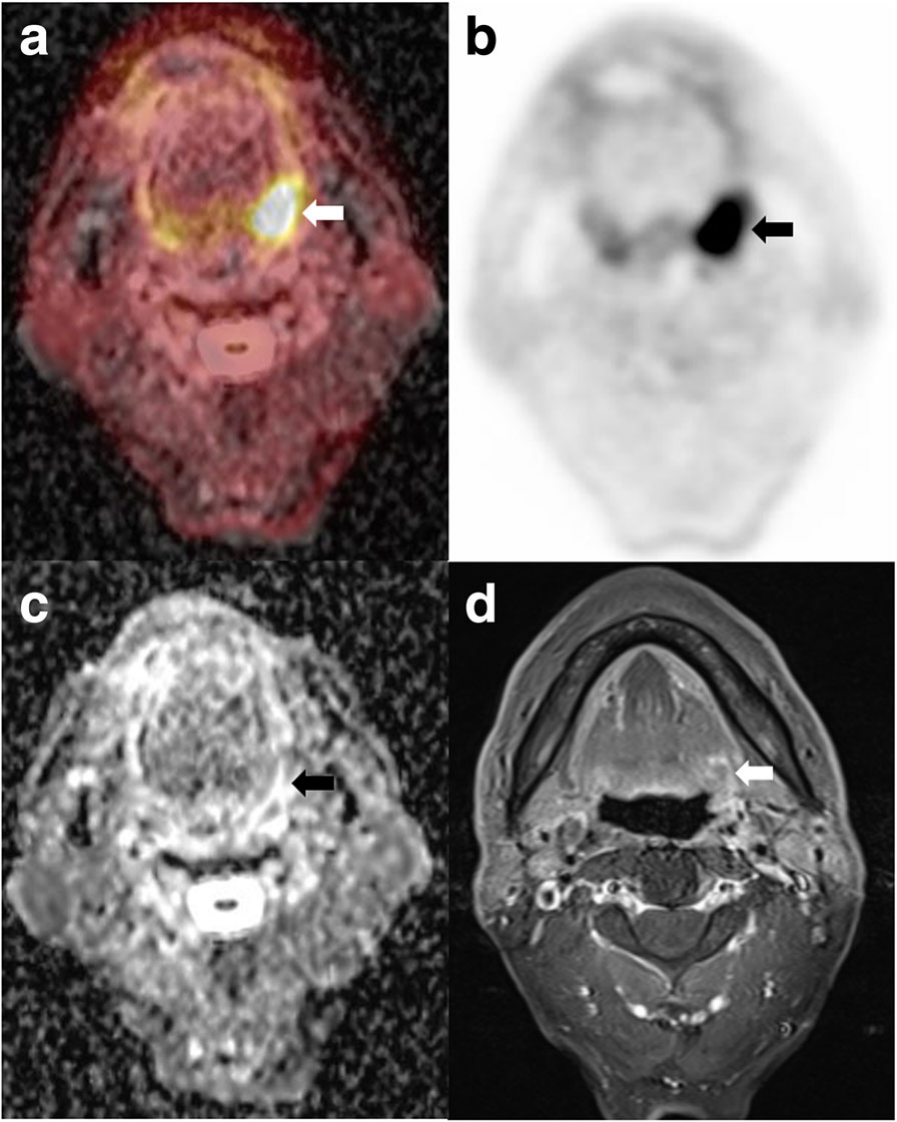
Case of tonsillar cancer with no evidence of disease predicted by combined PET/MRI parameters but treatment failure predicted by PET parameters. A 66-year-old male underwent preoperative simultaneous ^18^F-FDG PET/MRI due to a 3.8-cm-sized left tonsillar cancer. a Fusion image of regional PET with ADC map and b regional PET images showed left tonsillar mass with hypermetabolism (arrows). c ADC map image demonstrated left tonsillar mass with diffusion restriction (arrow). d Gadolinium-enhanced T1 axial image revealed mass with peripheral enhancement (arrow). ADCmean (1443.85), (SUVmax/ADCmean) × 1000 (10.37), (MTV/ADCmean) × 1000 (10.69), and (TLG/ADCmean) × 1000 (57.53) predicted no evidence of disease; however, SUVmax (14.97), MTV (15.44), and TLG (83.07) predicted treatment failure. The patient showed no evidence of disease until 41 months follow-up after tonsillar cancer surgery
